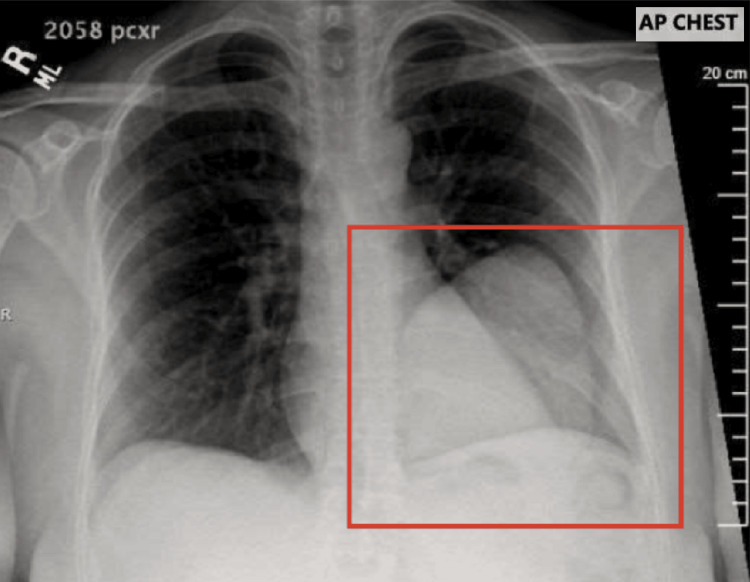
Chest x-ray with 9 cm cystic mass in the lower-left lobe
